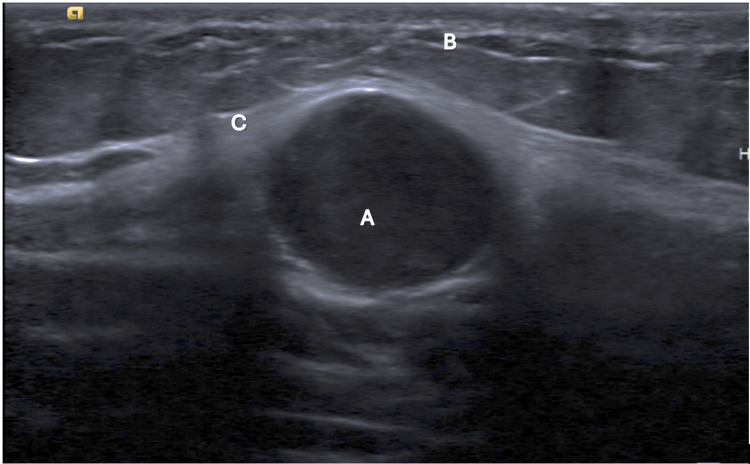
A preoperative abdominal soft tissue ultrasound of the mass Ultrasound demonstrates a nonspecific, hypoechoic 1.9 cm structure (A) in the subcutaneous tissue (B), positioned slightly deeper than expected for a typical epidermal inclusion cyst, i.e., deep to the fascia (C). Malignancy could not be excluded based on the ultrasound.
